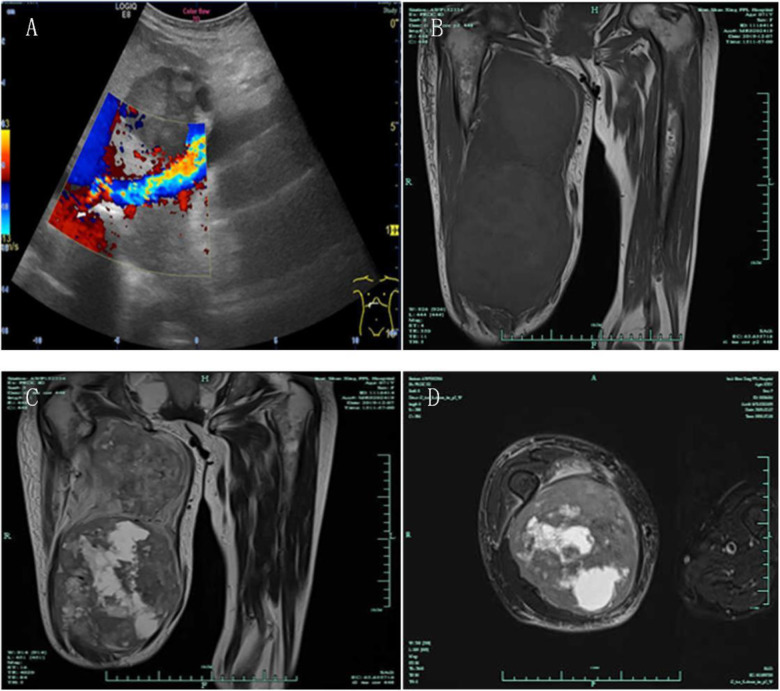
B-ultrasound showed that there was no deep vein stenosis or occlusion of the lower extremities, and the blood flow was unobstructed (A) MRI showed two masses with iso-signal intensity on T1-weighted images and hyper-intensity on T2-weighted images compared with the surrounding muscles ((B) T1 imaging, coronal plane; (C) T2 imaging, coronal plane; (D) T2 imaging, transverse plane).
